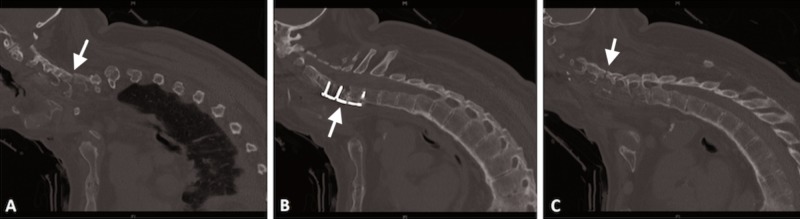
CT scan of cervical/thoracic spine after anterior cervical fusion CT scan of the cervical/thoracic spine demonstrating anterior cervical fusion C5-7 (B) with improvement of the alignment of fractured posterior elements (A, C). Note the kyphotic deformity.
